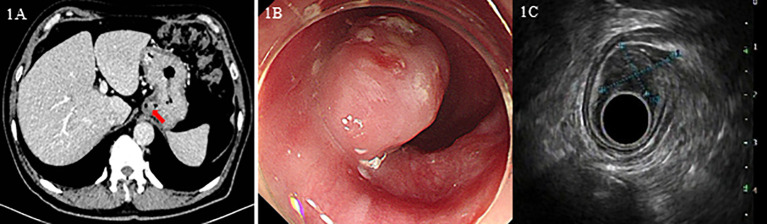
Multimodal imaging with CT, endoscopy, and endoscopic ultrasound(EUS) (A) Slight localized wall thickening of the lower esophagusis seen in the cross-sectional view of thoracicand abdominal enhanced CT; (B) shows the mucosal bulge near the cardia of the esophagus: (C) EUS revealed a well-demarcated, homogeneous hypoechoic mass originating from the submucosa.
